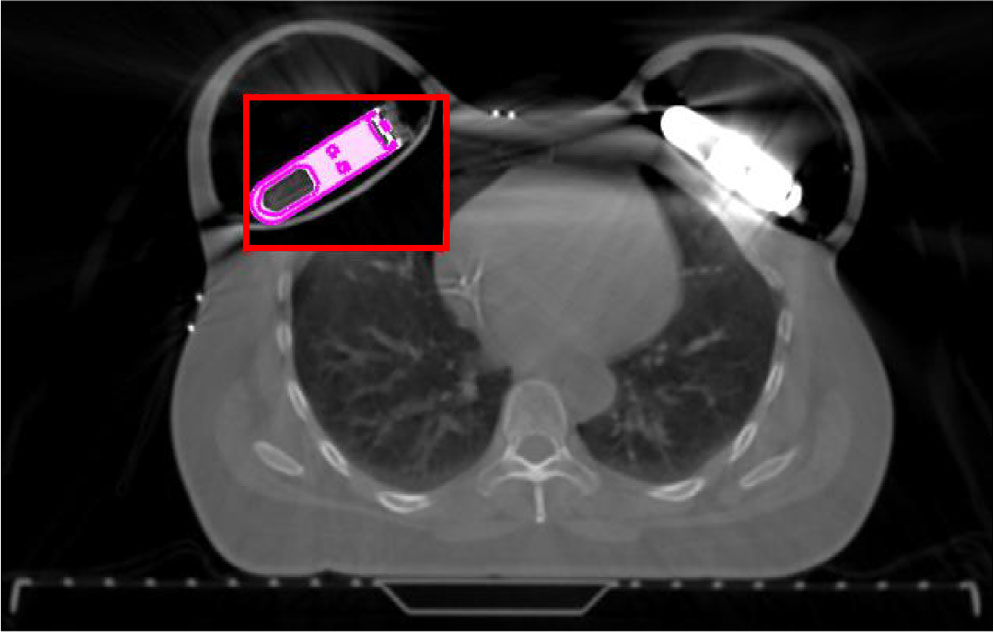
Bilateral AeroForm implants cause significant artifacts in the patient CT. Reservoir components are defined by registering the patient CT with to the treatment planning system model (shown in the “moving window”).
